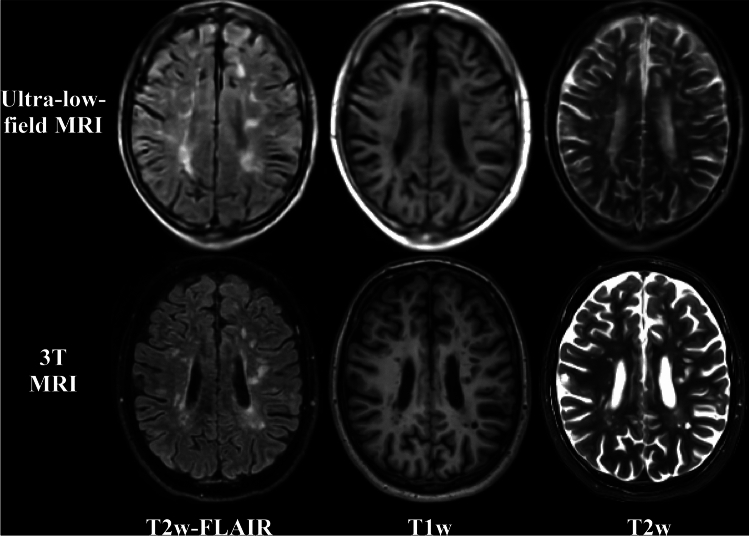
Ultra-low-field (ULF) and 3 T MRI in a 62-year-old male with late severe MS (EDSS 7.5). Figure shows native FLAIR, T2 and T1-weighted sequences on ULF (top row) and 3 T (bottom row) MRI. Due to different slice thicknesses and geometric distortions, perfect anatomical matching between the ULF and 3 T is infeasible
